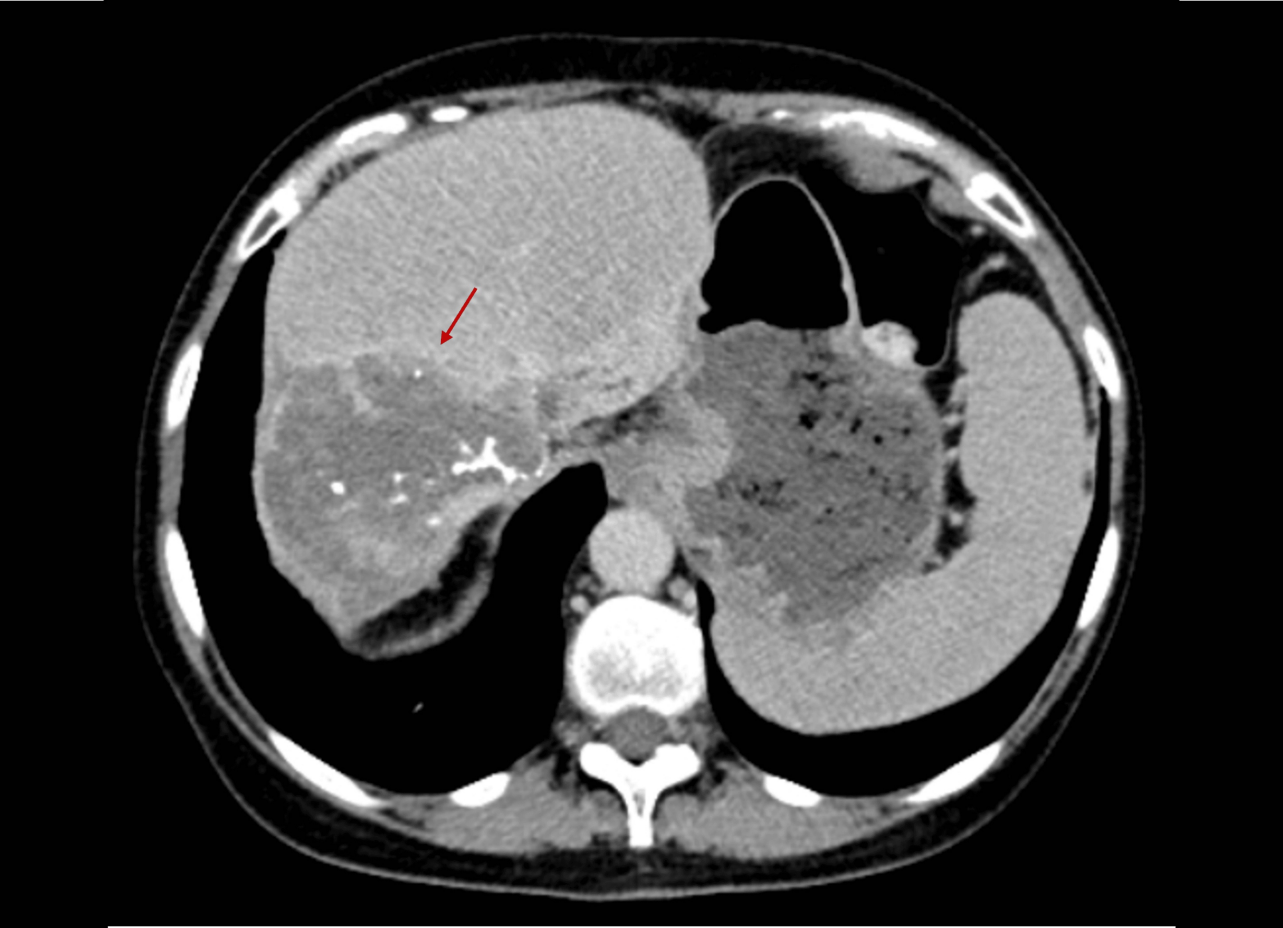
CT image before the patient was evaluated for liver transplantation (delayed phase) CT: computed tomography
